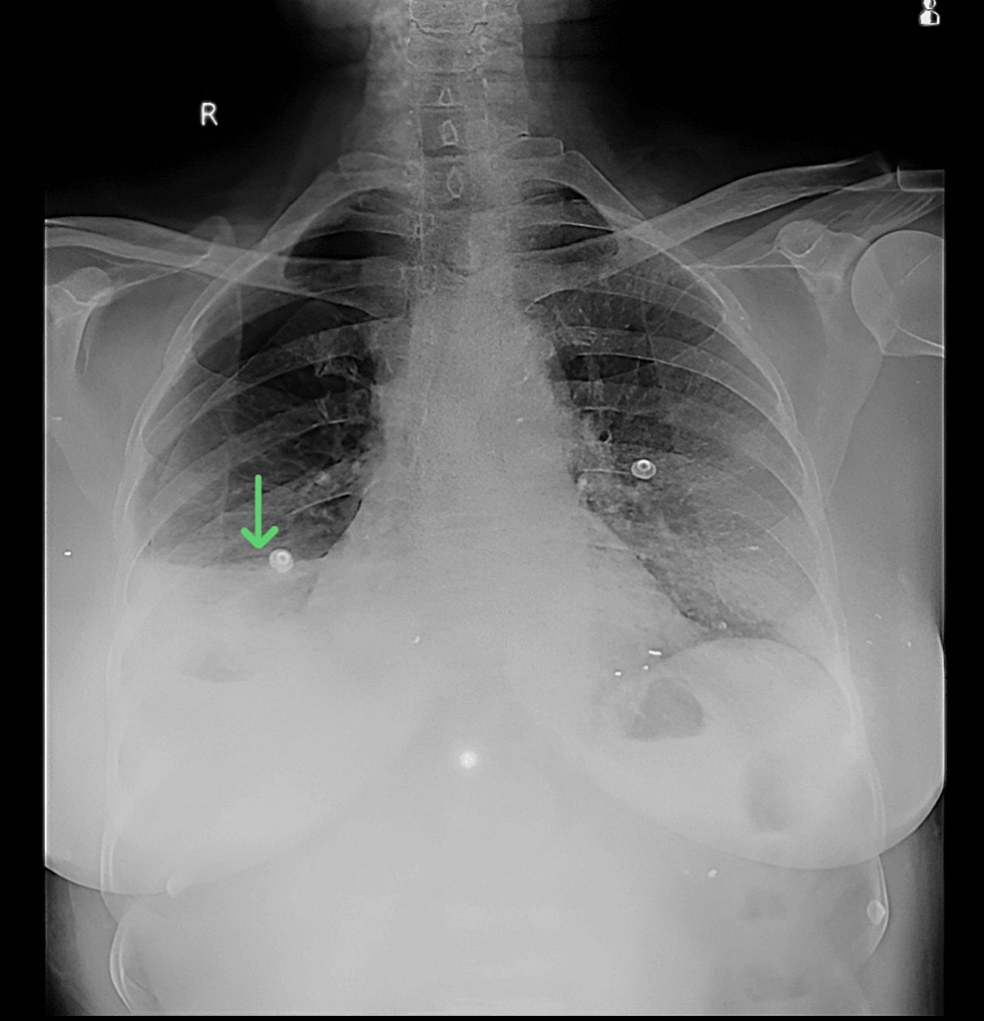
A supine anteroposterior chest X-ray showing right-side hydropneumothorax
